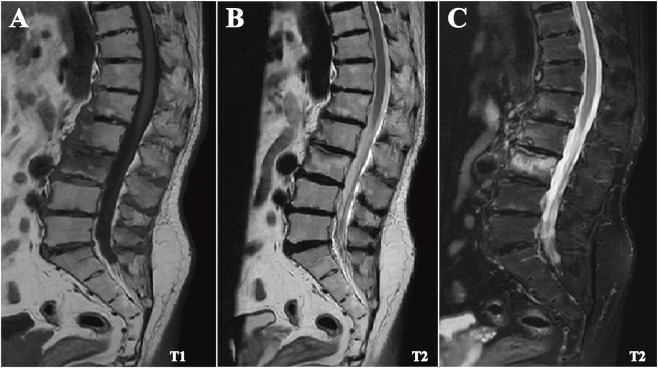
Lumbar spine MRI findings. (A–C) MRI of the lumbar spine demonstrating multilevel degenerative changes, including mild-to-moderate intervertebral disc space narrowing and reduced T2-weighted signal intensity of the discs, with thickening of the posterior longitudinal ligament. Mild anterior wedge deformities are observed at T11–L1, along with an L3 vertebral compression fracture. Fat-suppressed sequences show patchy hyperintensity within the L1-L3 vertebral bodies, consistent with bone marrow edema.
